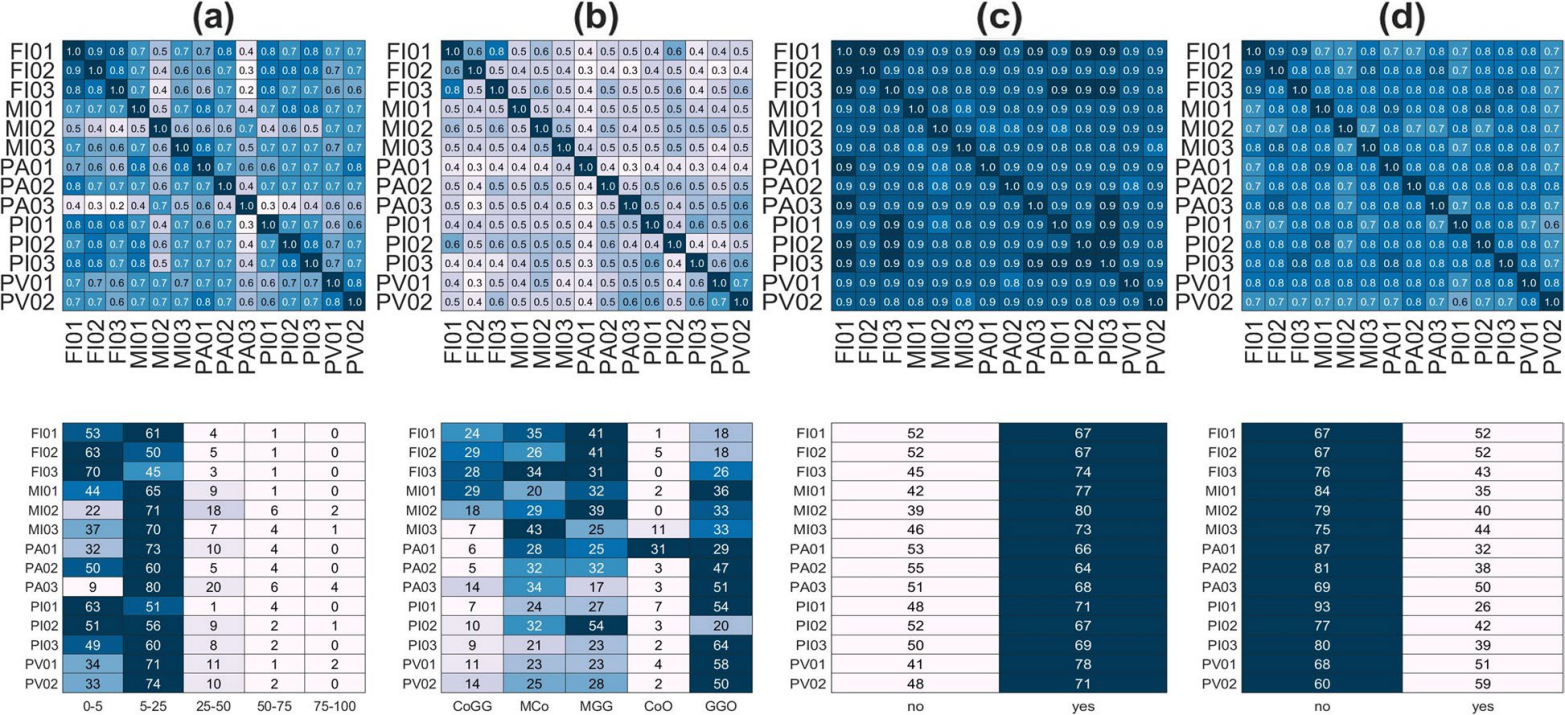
Top row: accuracy matrix on the four clinical metrics: computed tomography severity score (a), lesion type (b), bilateral (c), and basal predominant (d) lesion distribution. Bottom row: number of cases per reader and metric class
